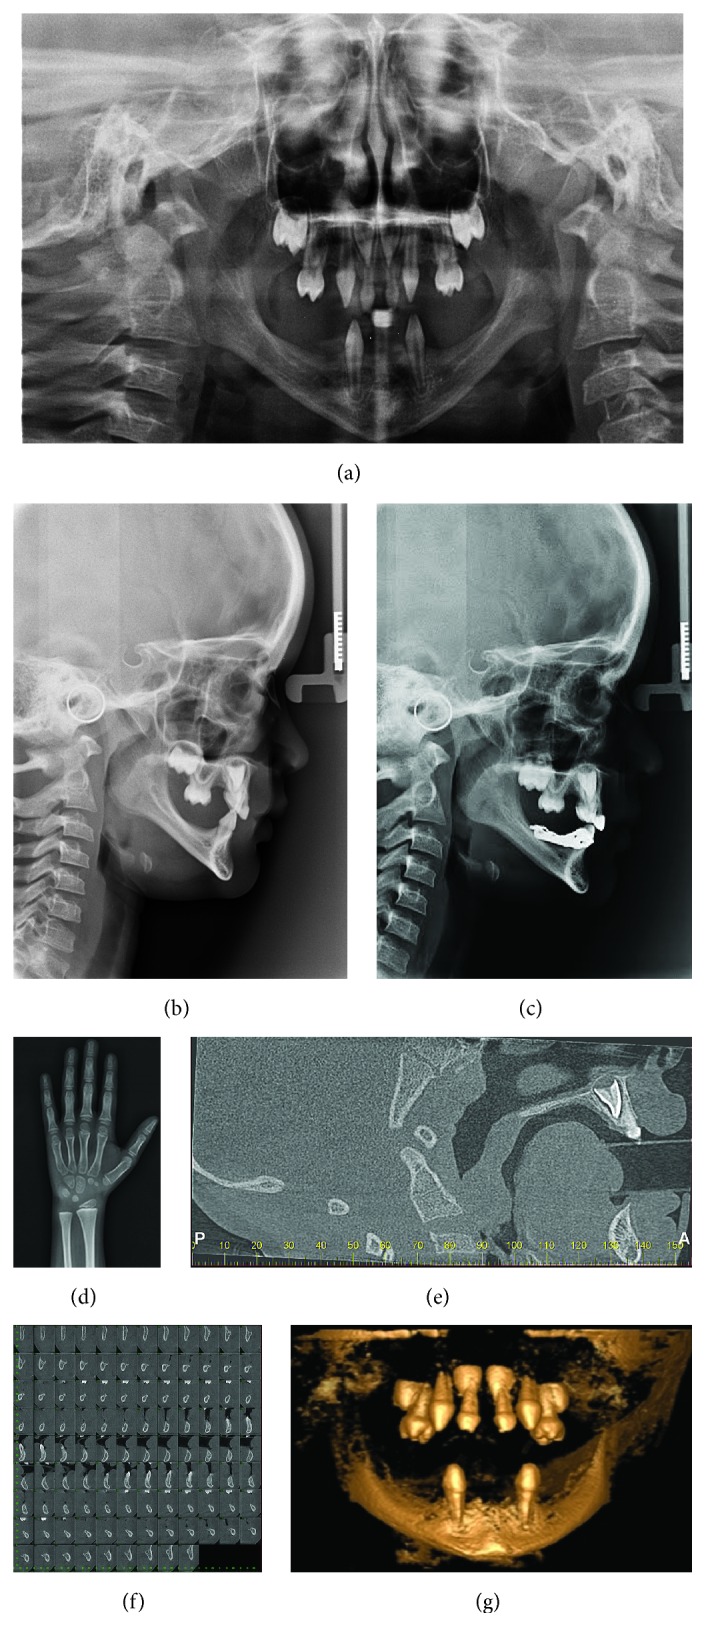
(a) Panoramic radiograph. (b) Lateral cephalogram showing decreased mandibular length and mandibular anterior facial height. (c) Lateral cephalogram taken 6 months after the patient began prosthetic rehabilitation. The image shows the growth pattern between the bone bases. (d) Carpal radiograph at 6 years of age matching the chronological age. (e) Computed tomography (CT) sagittal cut evidencing the presence of hypertrophic tonsils, consistent with recurrent infections of the lower respiratory tract. (f) Cross-sectional CT images showing reduced height and thickness of the alveolar bone, as well as mandibular micrognathia. (g) 3D reconstruction.
